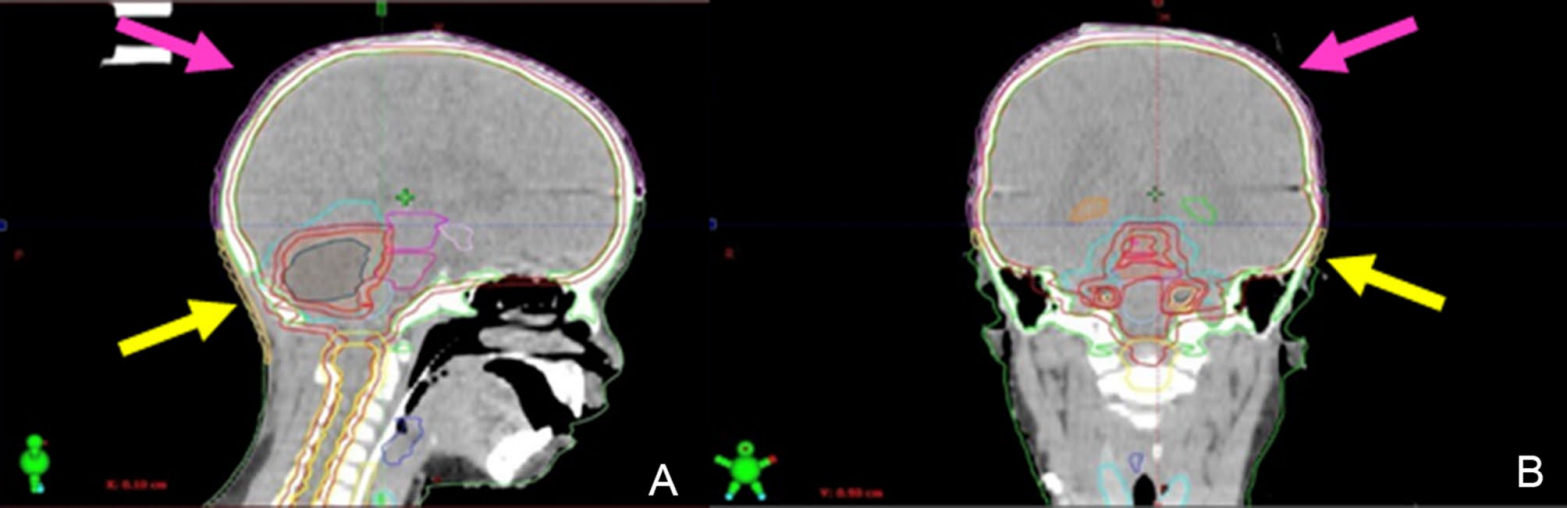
Original CT treatment plan scalp contouring. A Saggital and (B) Coronal view, whole-scalp ROI is pink colored and nape ROI is yellow colored. The thickness of skin contouring ranged from 3 to 5 mm based on the thickness of the skin. To determine the deep contour of the scalp we used the bone window, for the outer limit the soft tissue window
